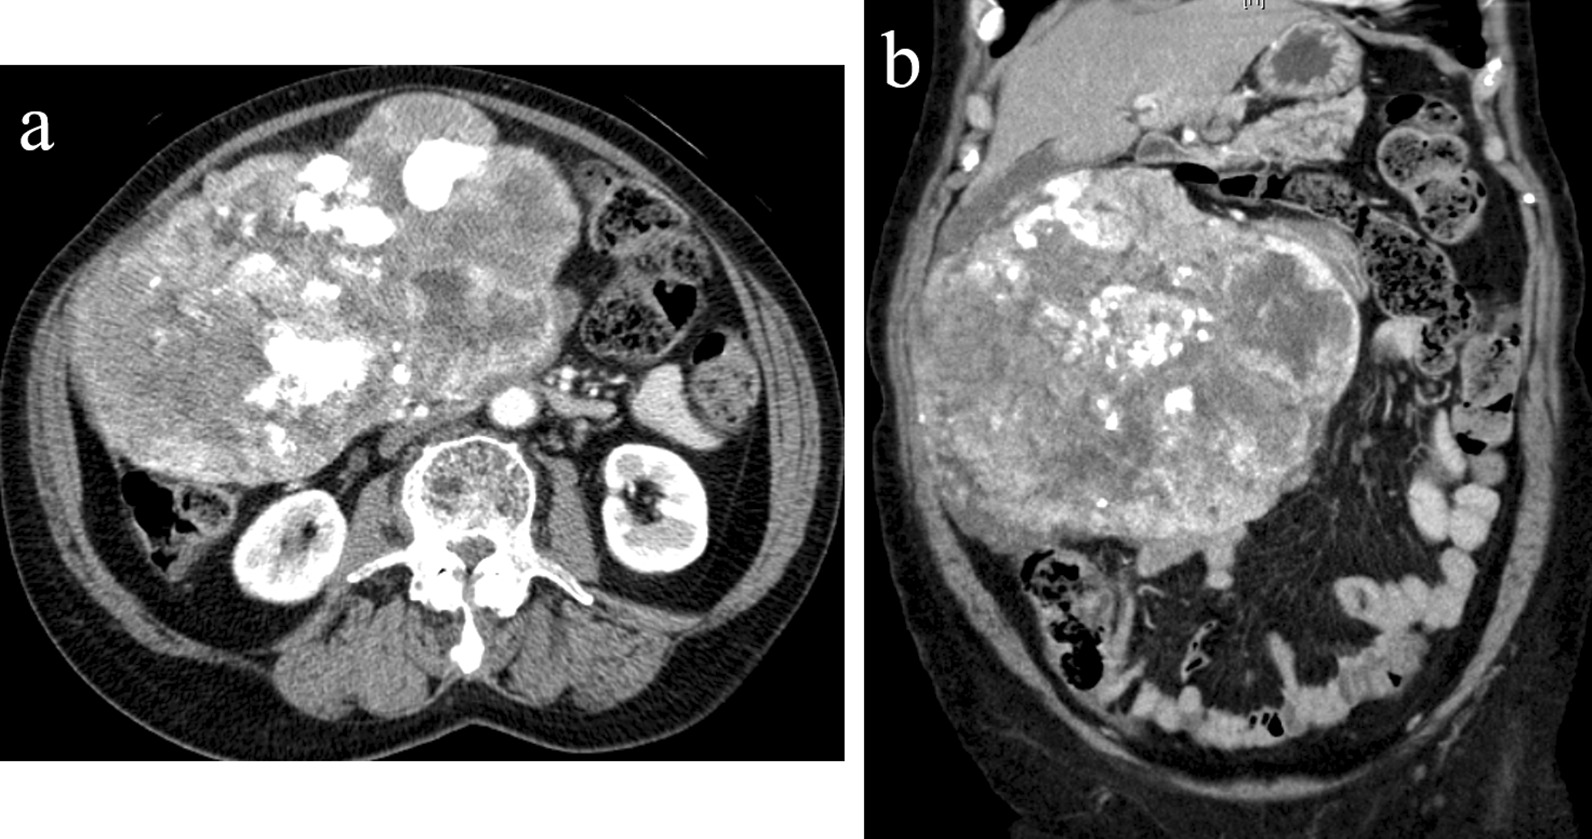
a, b Contrast-enhanced computed tomography showing that the tumor measured 25 × 22 cm, extended from the stomach, and abutted to the abdominal wall, liver, ascending colon, and abdominal aorta. The mass demonstrates heterogeneous contrast and internal low-density components
